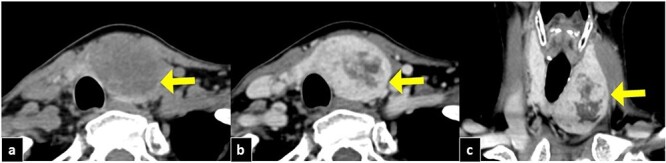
Cervical CT imaging. The tumor is well defined in the left lobe of the thyroid gland, with a size of 50 mm. (a) CT section in the axial section, showing a tumor with low density (arrow). (b) Axial section with gadolinium contrast (arrow). (c) Coronal section with gadolinium contrast (arrow).
